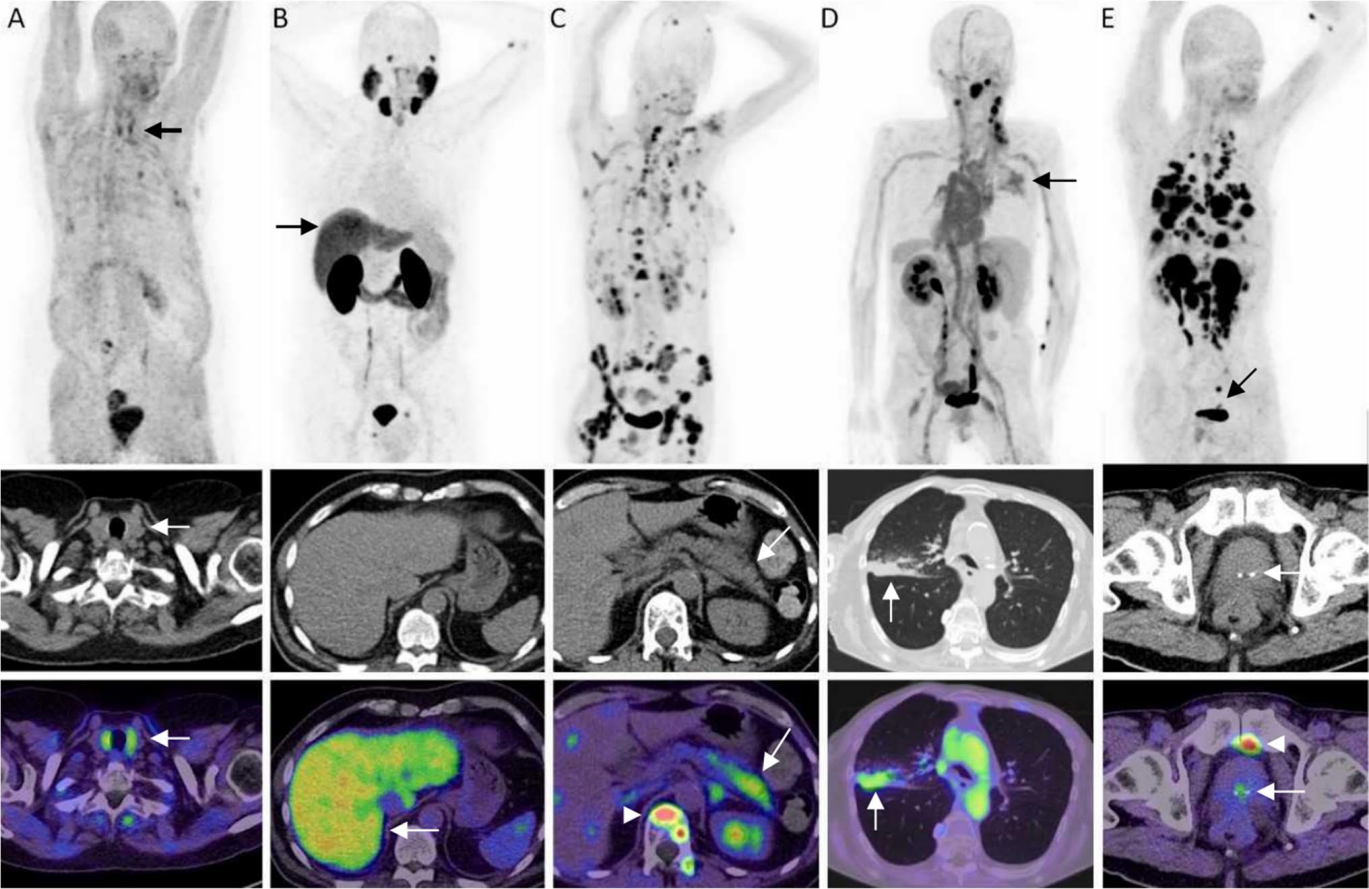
A A female 69-year-old patient with adenoid cystic carcinoma of the parotid gland showing a homogenous, high FAPI uptake of the thyroid with known Hashimoto thyroiditis (black arrow on MIP). B A 64-year-old male patient with esophageal cancer showing a diffuse, high uptake of FAPI of the whole liver (black arrow on MIP), while CT scan describes a nodular surface of the liver, concordant with fibrosis of the liver. A CT scan from January 2011 already described hepatic steatosis in this patient. C A 57-year-old patient with breast cancer showing a diffuse high uptake of FAPI in the corpus and tail of the pancreas (not shown on MIP, white arrow in transversal fusion image). A lab work performed 3 months after [^68^ Ga]-FAPI PET/CT scan showed slight elevated levels of LDH, potentially suggesting pancreatitis. Nonetheless, this differential diagnosis was not confirmed in further patient history. White arrowhead points to an osseous metastasis. D A 65-year-old patient with squamous cell carcinoma of the tongue and known chronic obstructive pulmonary disease showed an inflammatory pulmonary consolidation with an intense uptake of FAPI, which was deemed as pulmonary consolidation of the right upper lobe (black arrow on MIP, white arrow on transversal fusion image). E A 70-year-old patient with esophageal cancer showed a moderate uptake of FAPI in the prostate lobe (black arrow on MIP, white arrow on transversal fusion image) specifically in sight of a focal calcification of the prostate. A CT scan performed 1 month prior described a benign prostate hyperplasia (white arrowhead points to osseous metastasis in the pelvis).
